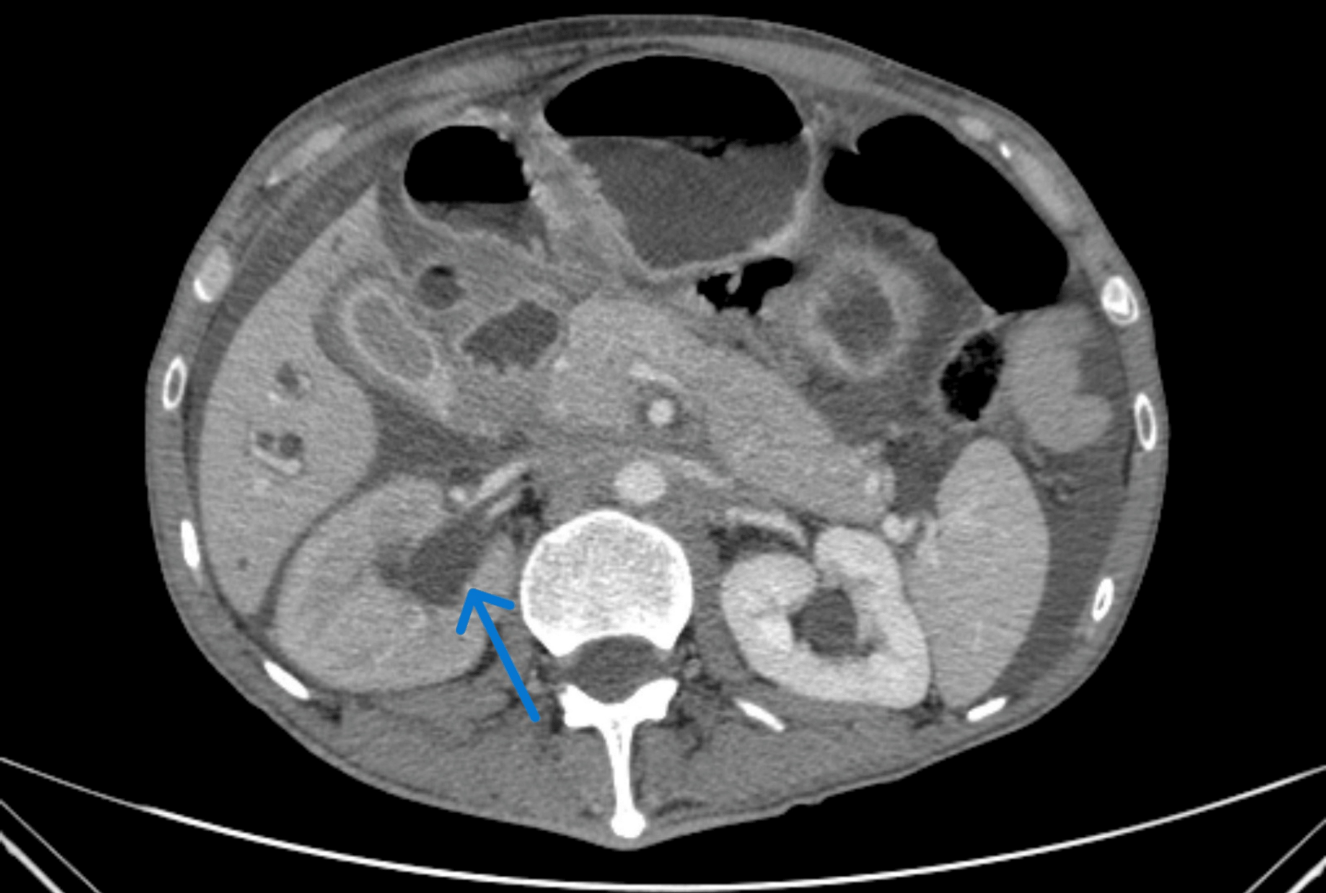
A contrast-enhanced CT of the abdomen and pelvis in September 2024 demonstrated a right hydronephrosis, thickening of the transverse colon, and gallbladder thickening with pericholecystic inflammation.
